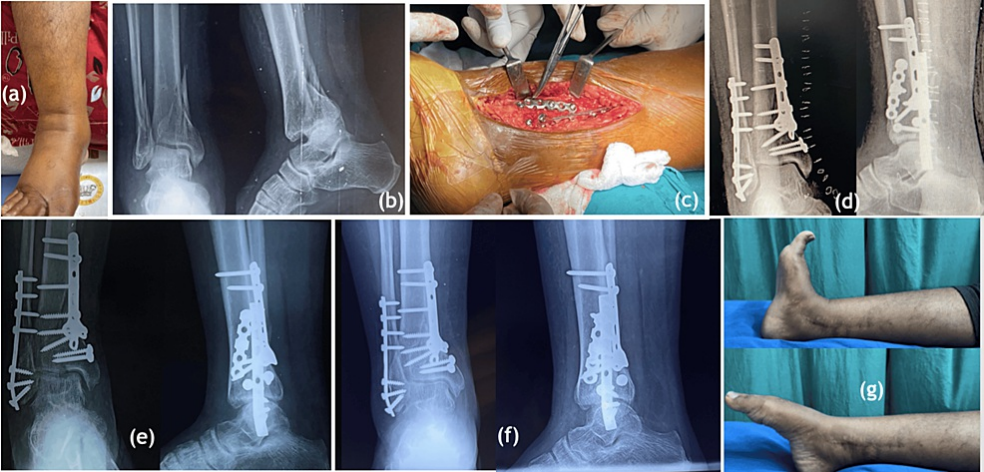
Patients with closed pilon fractures underwent internal fixation with excellent functional outcomes. (a) swollen ankle and foot, (b) AP and lateral radiographs on presentation, (c) internal fixation of the tibia through postero medial approach with Ellis plate and reconstruction plate, AP and lateral radiographs taken (d) on POD 2, (e) after 6 months of fixation, (f) after one year of fixation, (g) excellent ankle range of motion with dorsiflexion of 15° and plantar flexion of 35° AP: antero posterior; POD: post-operative day
